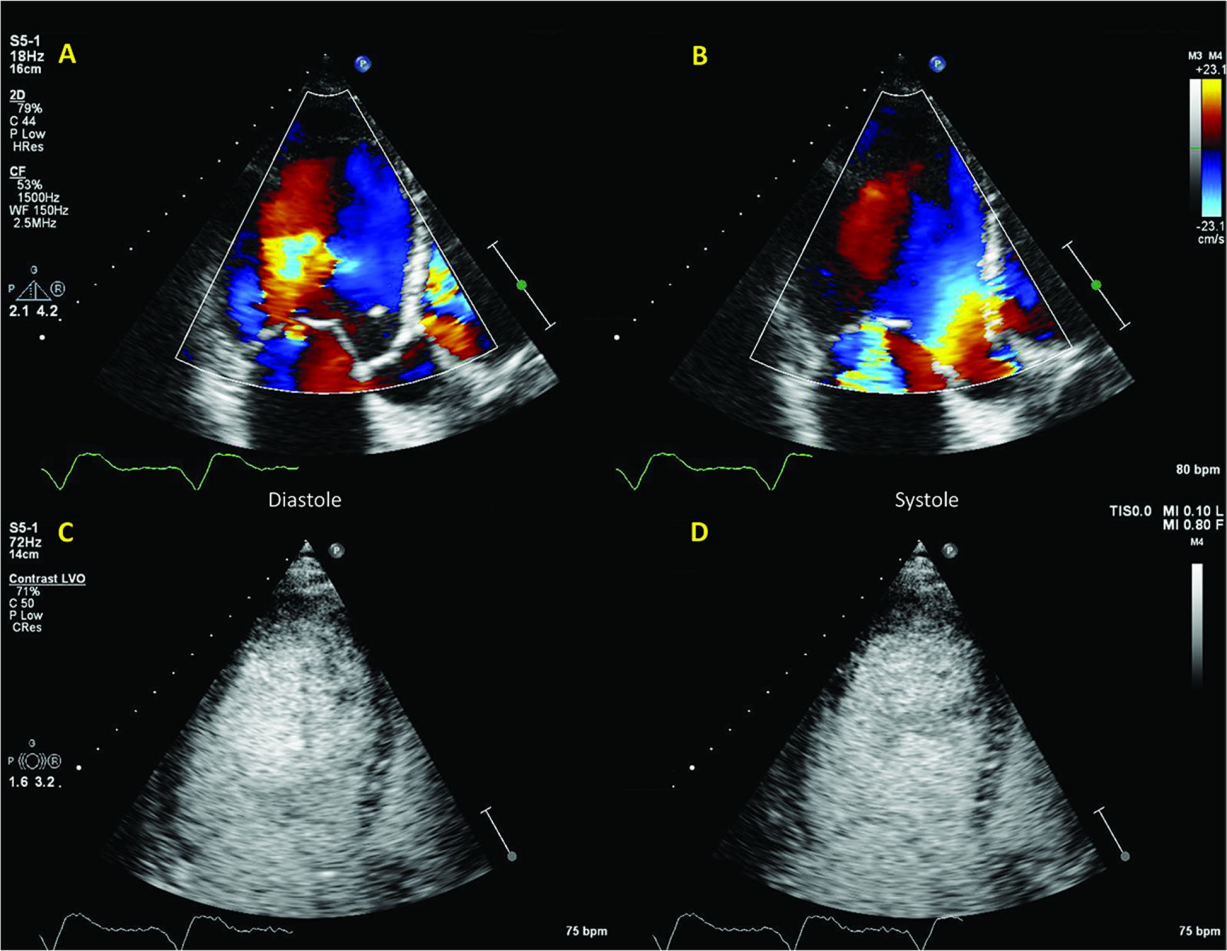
Patient 3. A, B: Color Doppler LV suggested clockwise rotating flow with low velocity (Additional file 9). Note the low color velocity scale, indicating low flow; C, D: Clinical LV contrast imaging demonstrated the same rotational flow, with apical swirling (Additional file 10)
